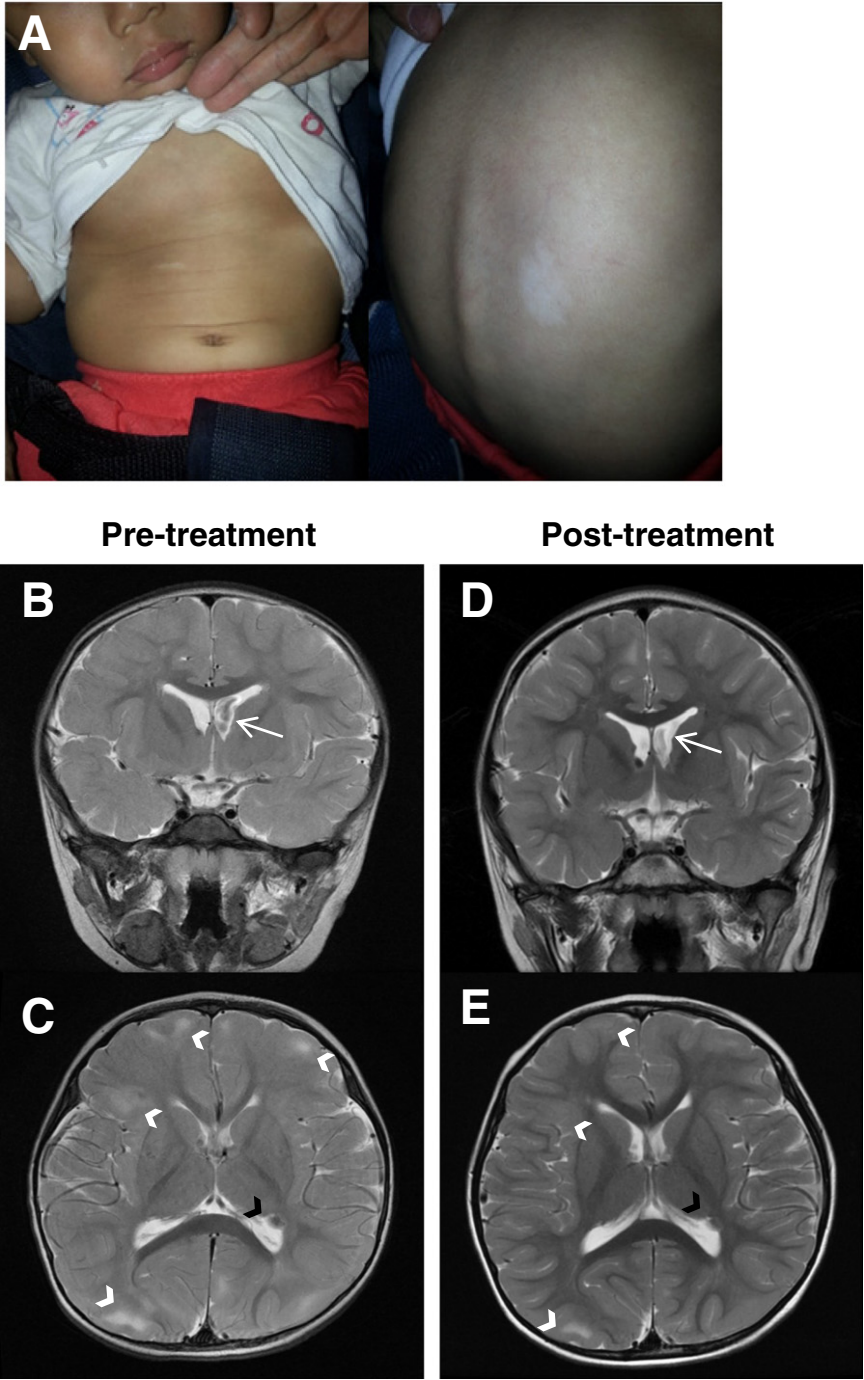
Cutaneous features and brain MRI findings of the patient. a Photographs showing several hypopigmented macules on the chest and abdomen (*left*) and on the back (*right*) of the patient. b-c. Pre-treatment brain MRI revealing a SEGA (*white arrow*) located at the foramen of Monro within on coronal T2-weighted imaging (b), multiple cortical tubers (*white arrowheads*) and a subependymal nodule (*black arrowhead*) within on axial T2-weighted imaging (c). d-e Post-treatment brain MRI demonstrating size reduction of the SEGA (*white arrow*) (d), reduced cortical tubers (*white arrowheads*) and the same size of subependymal nodule (*black arrowhead*) (e) compared to pre-treatment MRI
